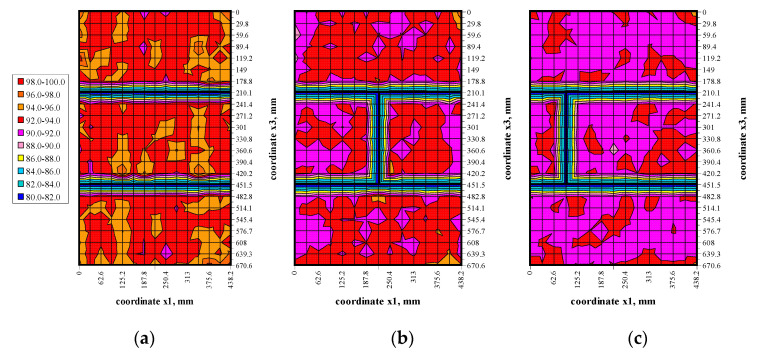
Results from measuring passing time of the ultrasonic wave under the load *σ*_3_ = 0.50*σ*_3max_: (a) model I-1, (b) model II-1, (c) model III-1.
